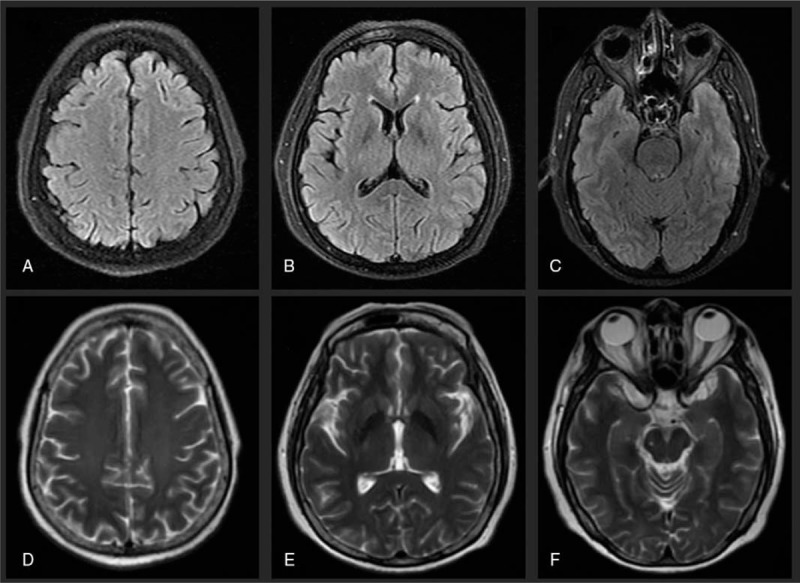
Case 1, 10 days later after treatment initiation, MRI showed resolution of parieto-occipital lesions on FLAIR (A–C) and T2-WI (D–F).
